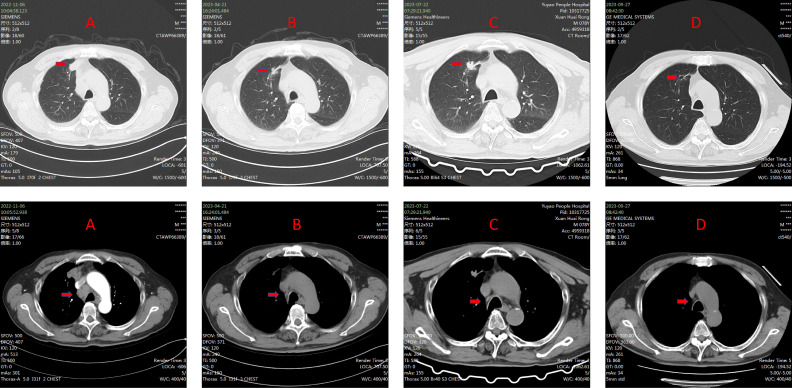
(A) The computed tomography (CT) scan before treatment. (B) the CT scan after 6 cycles of administration of tislelizumab, showing that the tumor significant reduction. (C) The CT scan after 8 cycles of administration of tislelizumab, showing that the tumor had increased in size. It indicates that the tumor has developed resistance to tislelizumab. (D) the CT scan conducted two months after the administration of savolitinib showed that the tumor shrank again.
